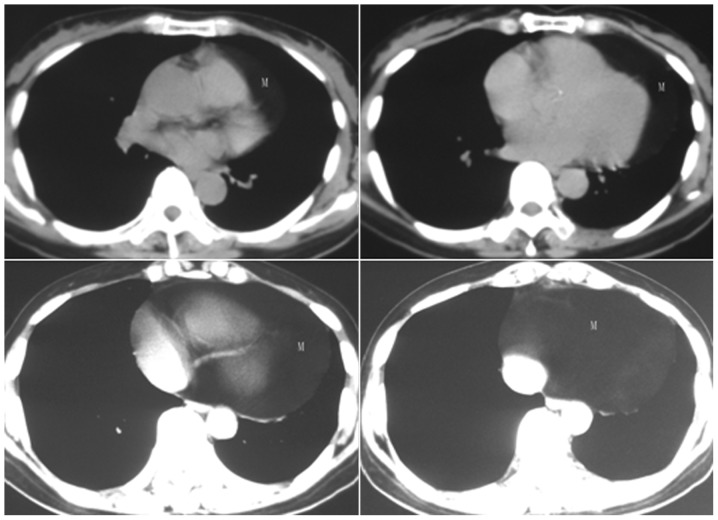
Multi-detector computed tomography plain scan images demonstrating a large lipoma in the anterior mediastinum surrounding the heart. The attenuation value of the mass was equal to that of subcutaneous adipose tissue. M, mass.
